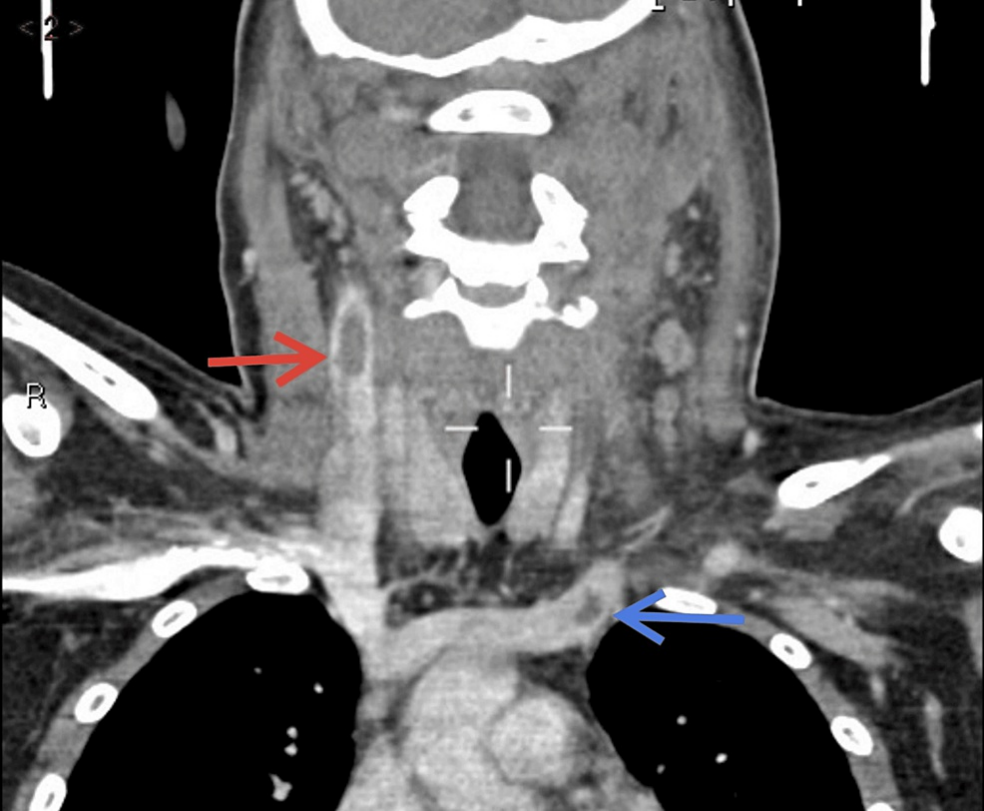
Contrast CT of the neck coronal view. The red arrow indicates a filling defect (thrombus) in the right internal jugular vein. The blue arrow indicates a filling defect (thrombus) at the junction of the left innominate and left internal jugular veins.
